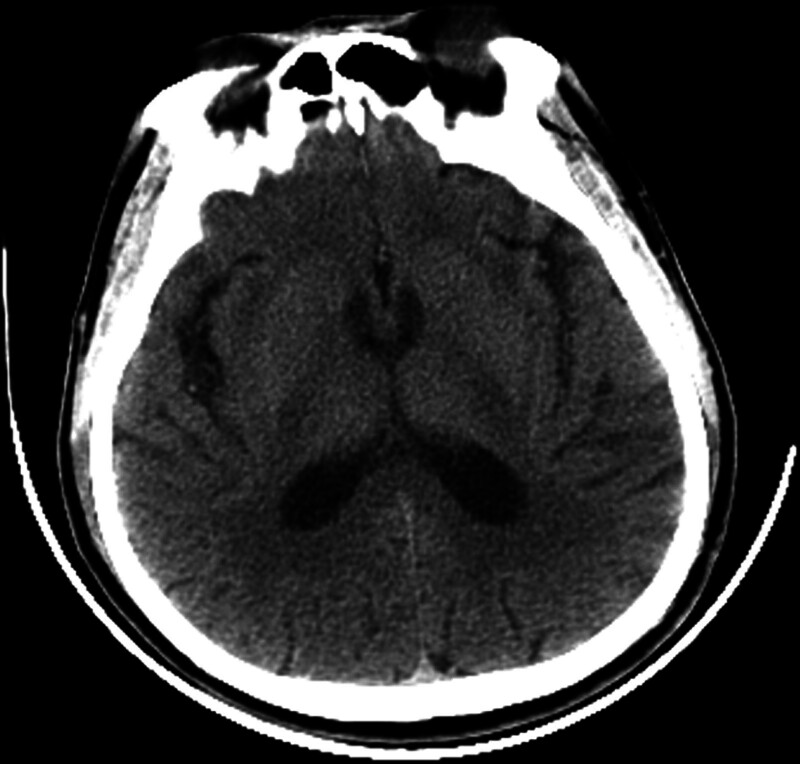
A CT scan of the head on the second day after the onset of the disease showed no abnormal signals. CT = computed tomography.
